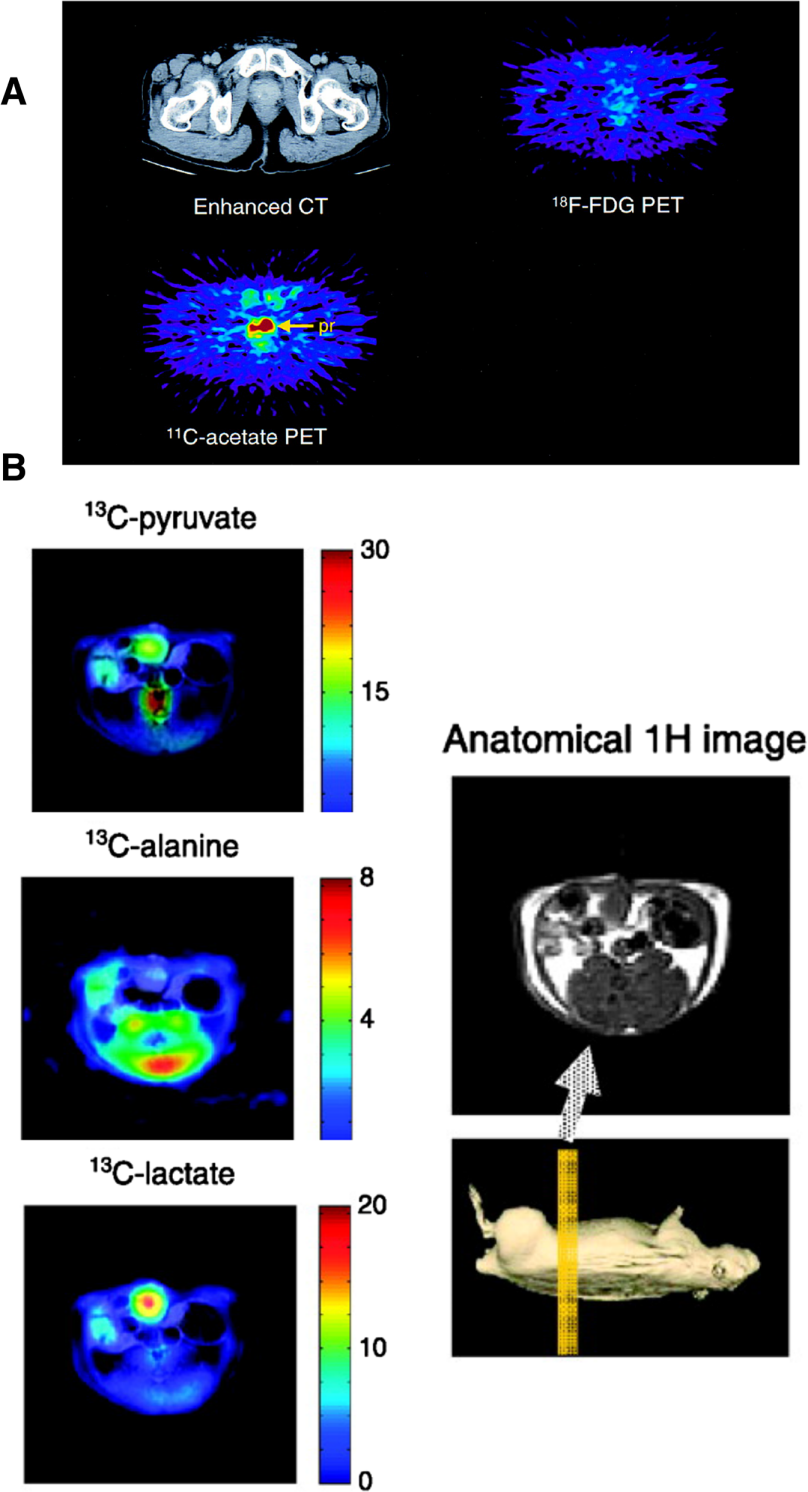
a PET/CT images comparing [^11^C] -acetate and FDG in a prostate cancer patient. This research was originally published in the Journal of Nuclear Medicine. Oyama N, Akino H, Kanamaru H, Suzuki Y, Muramoto S, Yonekura Y, Sadato N, Yamamoto K, Okada K: ^11^C-acetate PET imaging of prostate cancer. J Nucl Med 2002, 43:181-186. © by the Society of Nuclear Medicine and Molecular Imaging, Inc [58]. b Hyperpolarized MR imaging of hyperpolarized [^13^C] pyruvate and its conversion to [^13^C] alanine and [^13^C] lactate in vivo. Adapted by permission from the American Association for Cancer Research: Golman K, Lerche M, Pehrson R, Ardenkjaer-Larsen JH: Metabolic imaging by hyperpolarized 13C magnetic resonance imaging for in vivo tumor diagnosis. Cancer Research 2006, 66:10855-10860 [58]
